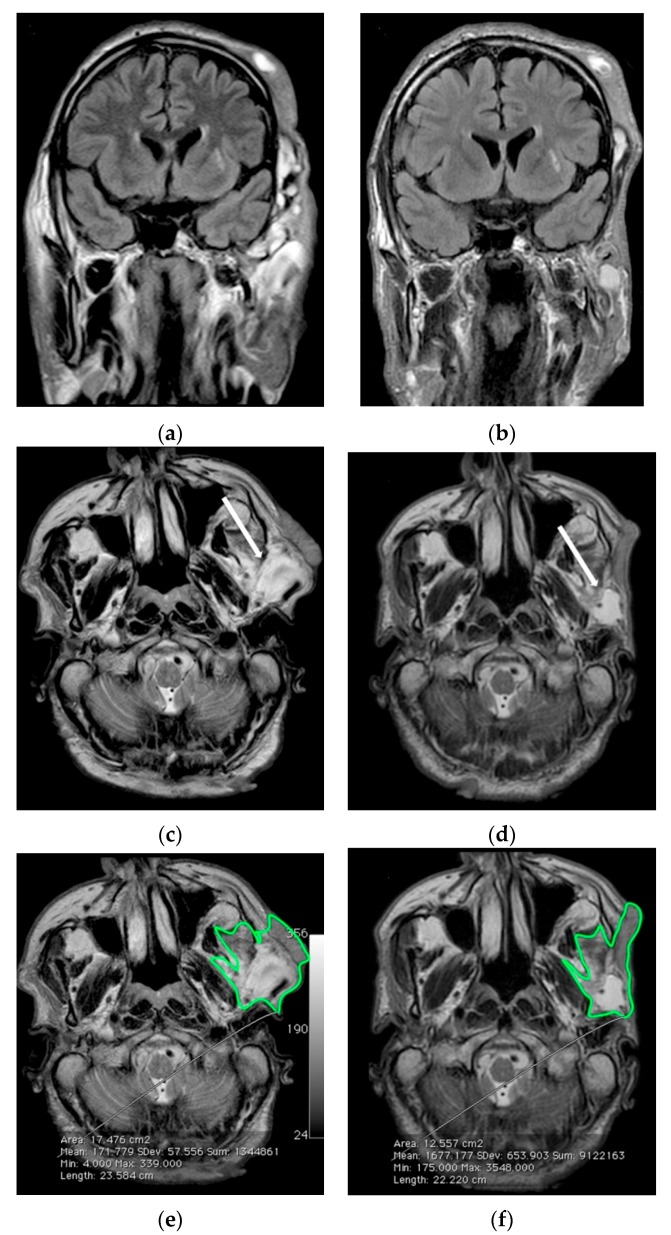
Serial MR imaging assessment of case 11. (a) Coronal FLAIR-weighted and (c,e) Axial TSE T2-weighted images of the left facial plexiform neurofibroma at baseline; (b) Coronal FLAIR-weighted and (d,f) Axial TSE T2-weighted images after six months of MedDietCurcumin. After six months there was a significant reduction especially in the hyperintense parts of the lesion, as indicated by arrows in (c,d). In (e,f) a green border contours the area used for volume measurement.
